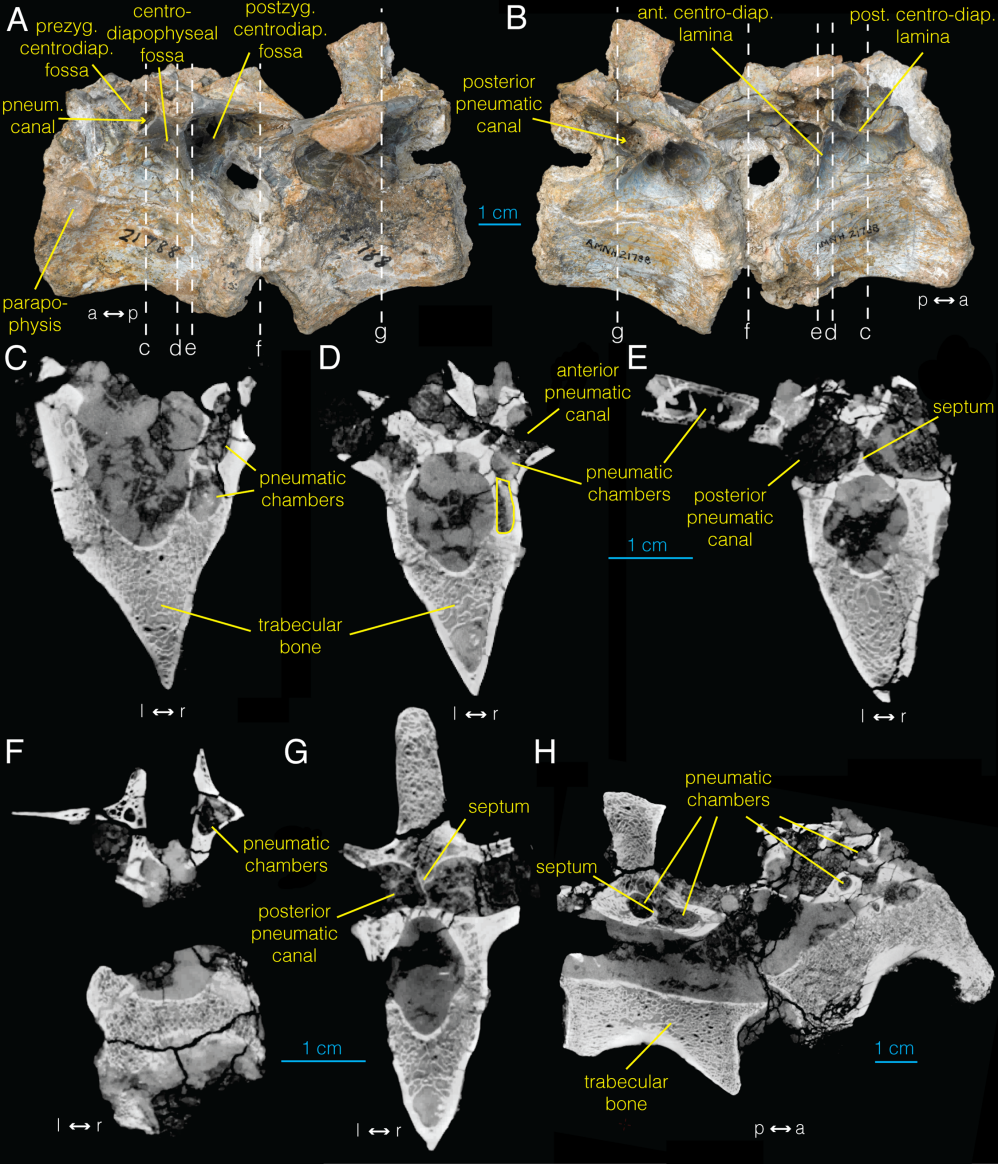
Anterior dorsal vertebrae of *Archaeornithomimus* (AMNH FARB 21788) and associated CT images. A, left lateral view; B, right lateral view; C–G, select transverse sections; H, midsagittal section. Dashed lines and associated letters indicate location and letter designation of CT image slices.
